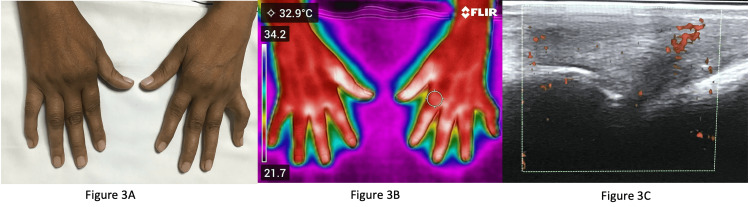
Image of a patient, whose hands were affected with rheumatoid arthritis (RA) 3A: Clinical image of a patient with long-standing RA; 3B: Infrared thermography of the same patient showing increased temperature over the right and left first, second, and third metacarpophalangeal (MCP) and proximal interphalangeal (PIP) joints; 3C: Ultrasonography findings of the same patient showing synovitis with power doppler uptake at the MCP joint.
